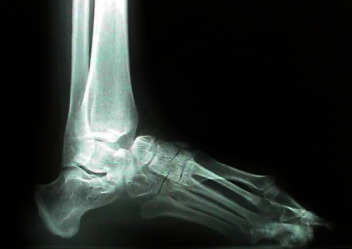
X-ray of the left foot, showing the horizontal alignment of the first metatarsal, flexion of the first MTP and an extension of the IPP joint of the hallux [2].
